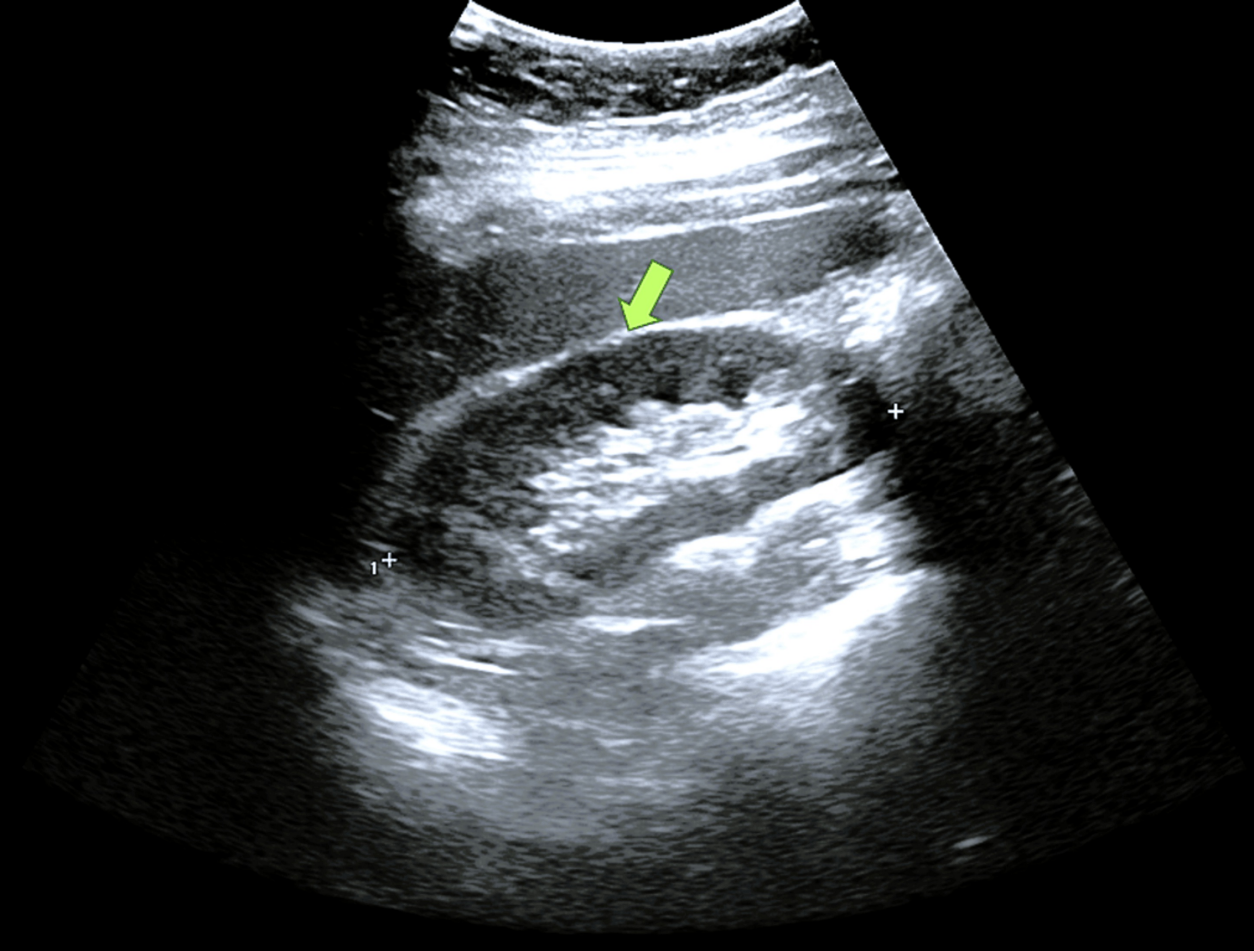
Follow-Up Ultrasound Showing Resolution of Hydroureteronephrosis Follow-up ultrasound image of the right kidney demonstrating complete resolution of previous hydroureteronephrosis (green arrow) with no residual calculus or back-pressure changes one week after spontaneous stone passage.
